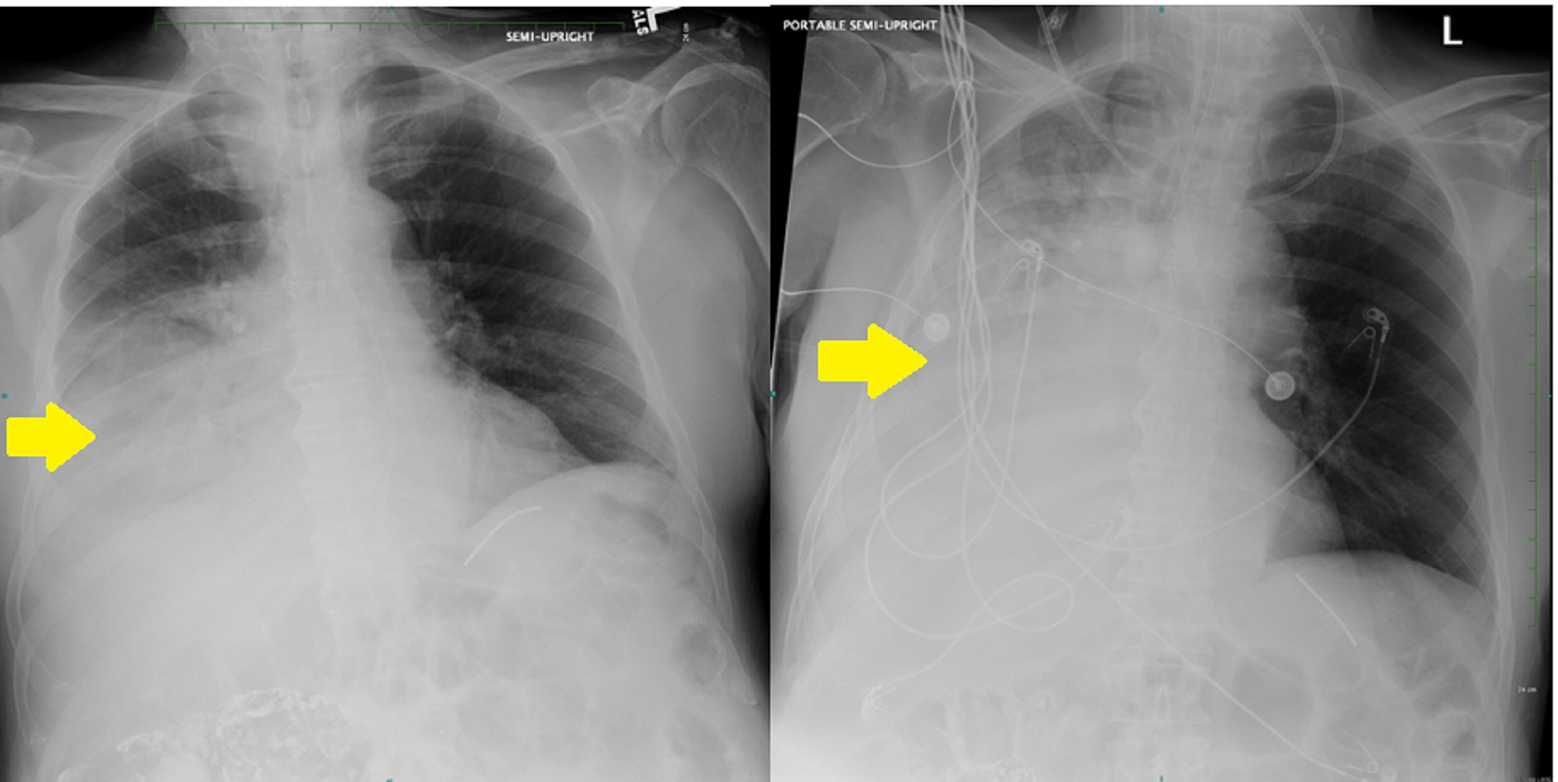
Sequential chest X-rays demonstrating progressive accumulation of right-sided pleural effusion (yellow arrows).
